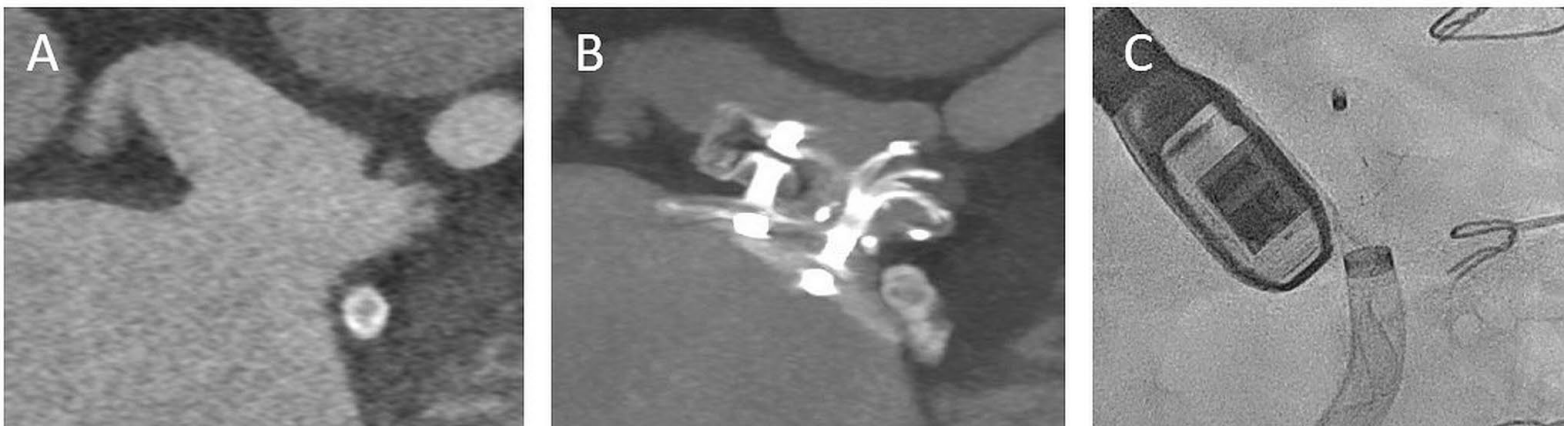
CT-aided planning for complex LAA intervention. An 82-year-old patient admitted for transcatheter left atrial appendage closure due to bleeding events. A Complex LAA anatomy with a large atypical orientated lobe in direction of the left atrial roof and a smaller LV orientated side lobe. B Intervention using a steerable sheath with 180° inverted implant. C After the initial procedure, a small posterior leak was successfully closed with a second occlude
